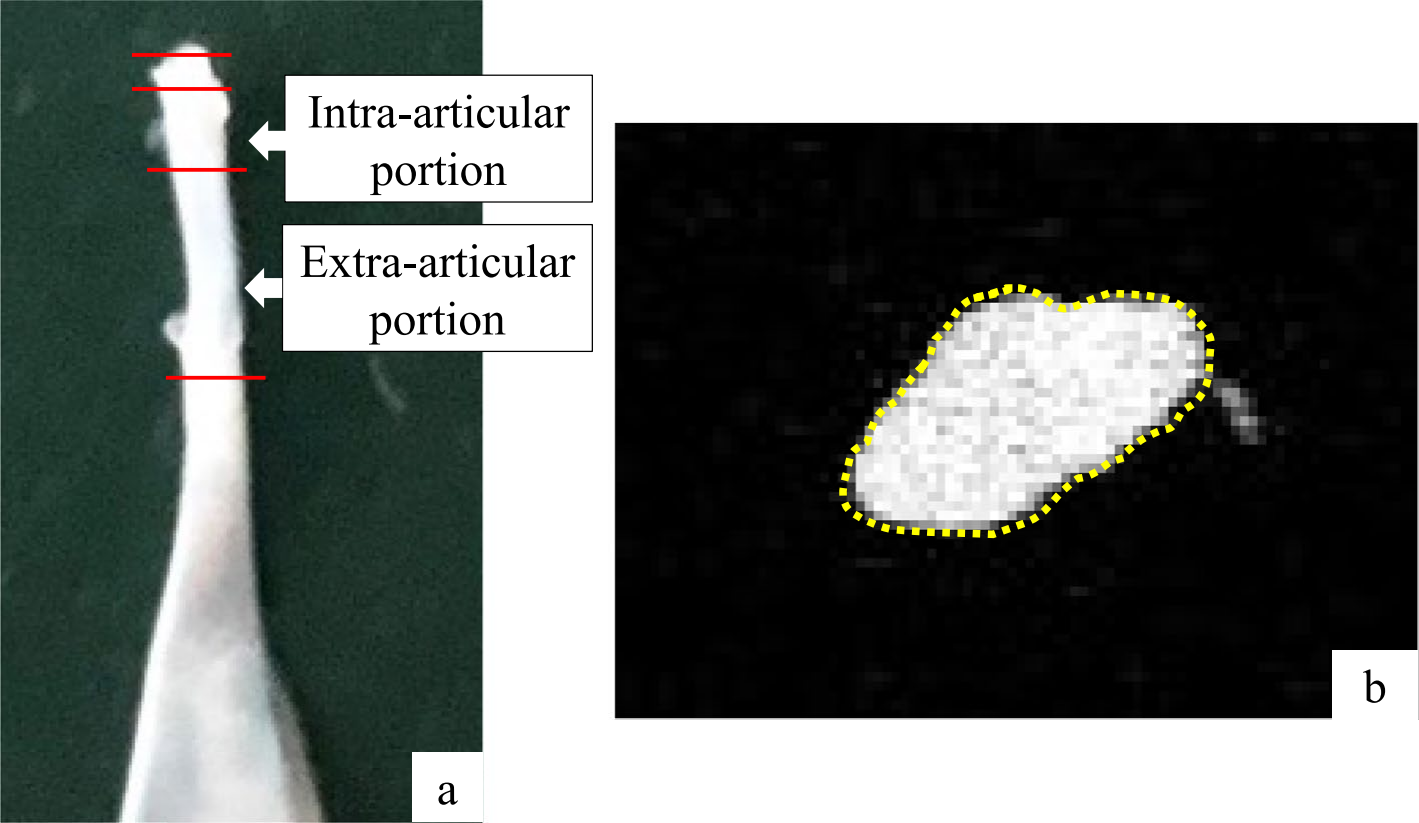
LHBT imaging (left shoulder). a Harvested LHBT. b Cross-sectional micro-computed tomography image of the LHBT. *LHBT* long head of the biceps tendon
